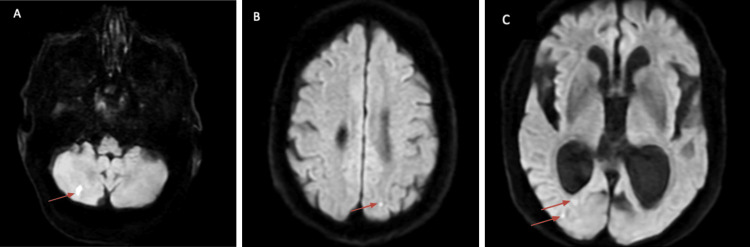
MRI of the brain without contrast demonstrating acute infarcts. (A) Acute right cerebellar infarct. (B) Acute left occipital infarct. (C) Acute right occipital infarcts.
